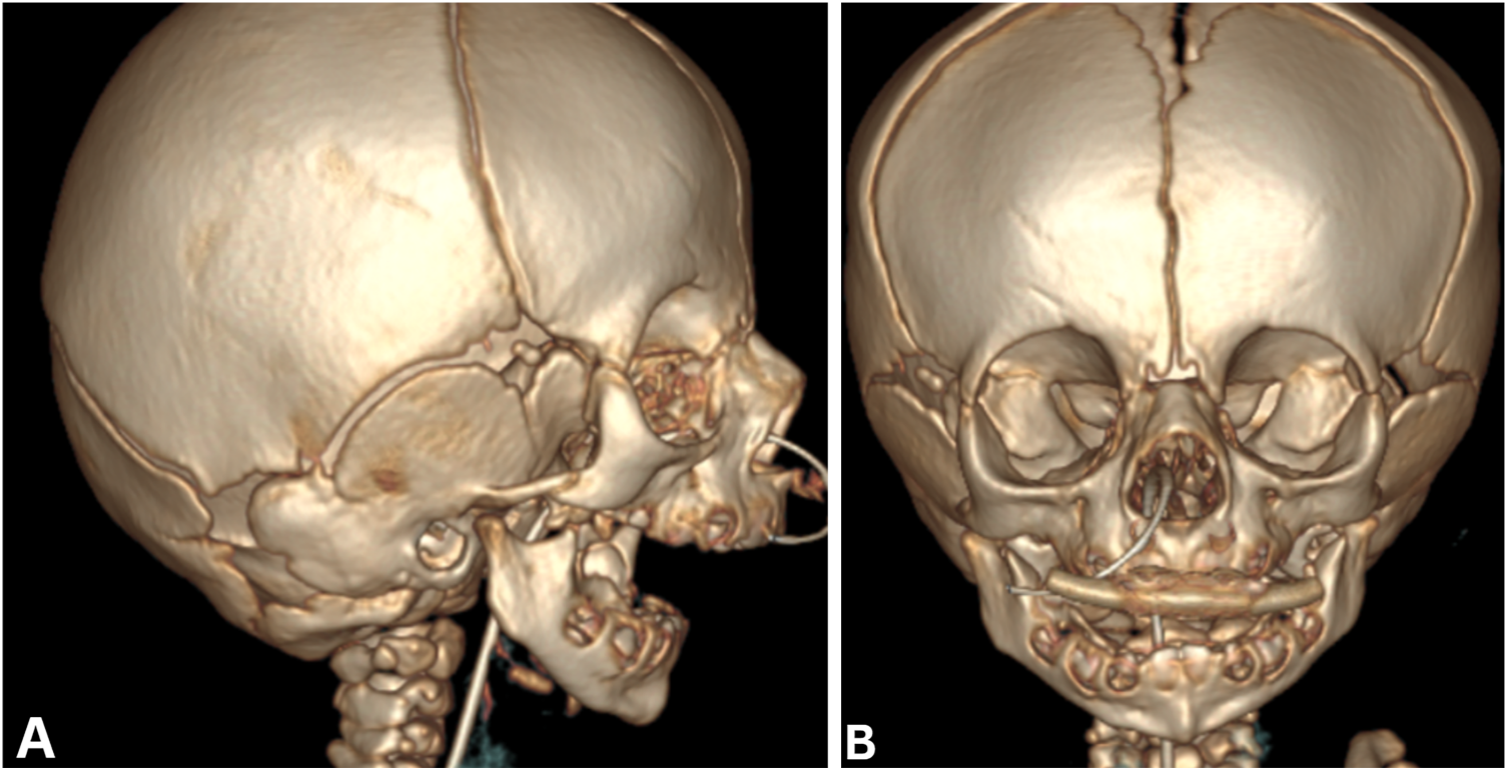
Computed tomography image reconstruction: lateral (A), anterior (B) demonstrating severe micro-/retrognathia.
